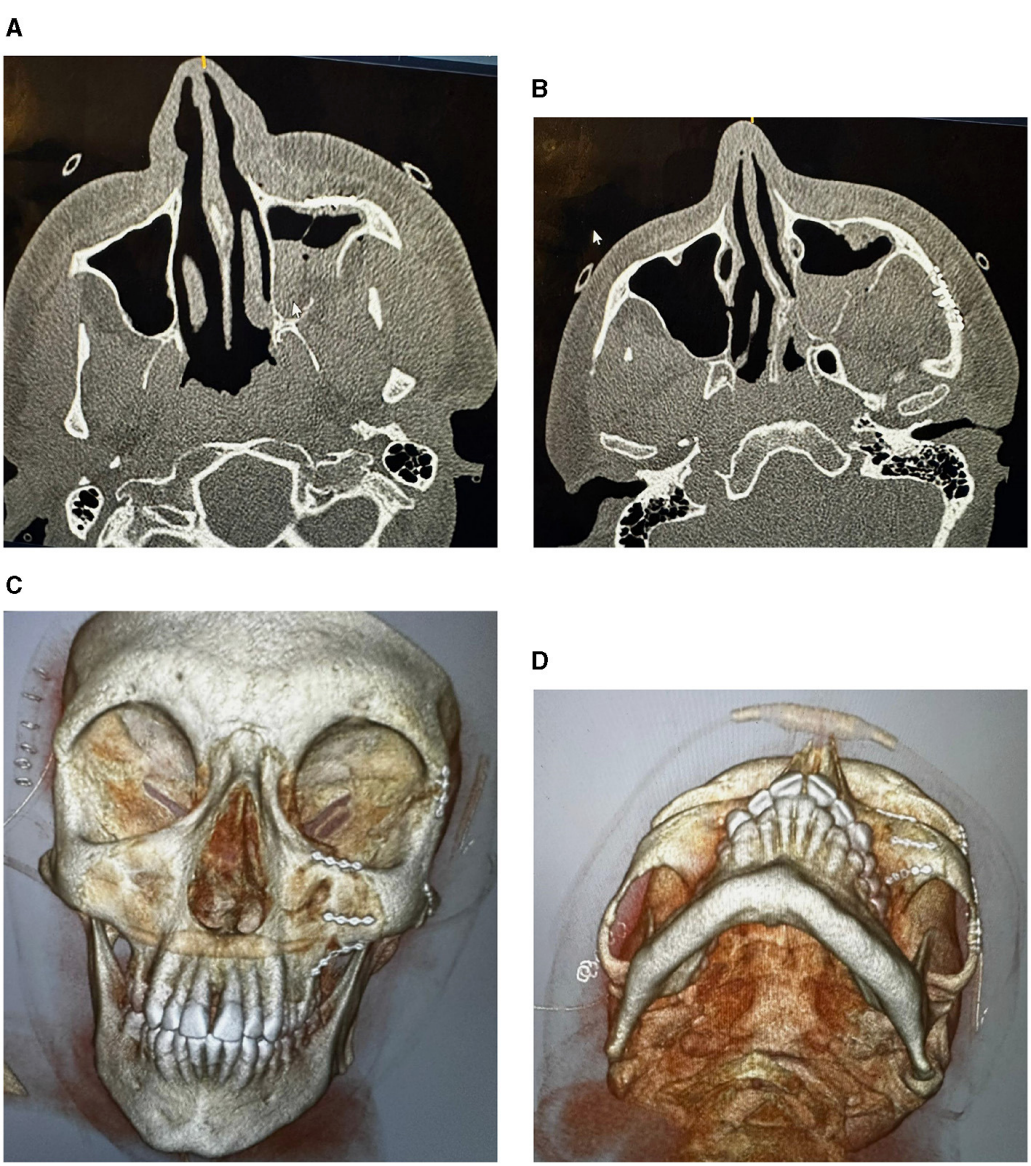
CT images showing factures of cheek and socket bones: before treatment (A), and after reduction and fixation (B–D).
